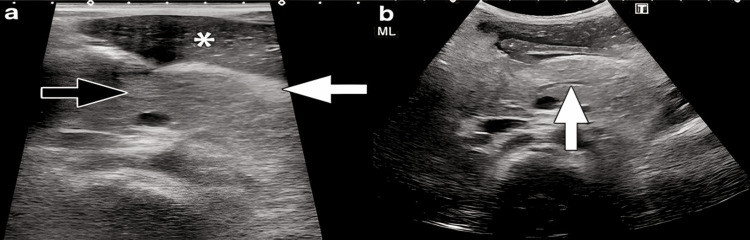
Transverse ultrasound images of the pancreas in a three-year-old boy with acute pancreatitis. Ultrasound was performed two days after the onset of an acute pancreatitis episode. a) The ultrasound shows a mildly bulky pancreas (black arrow) and echogenic, thickened peripancreatic fat reflecting edema (white arrow). b) Dilatation of the main pancreatic duct in the body of the pancreas.
